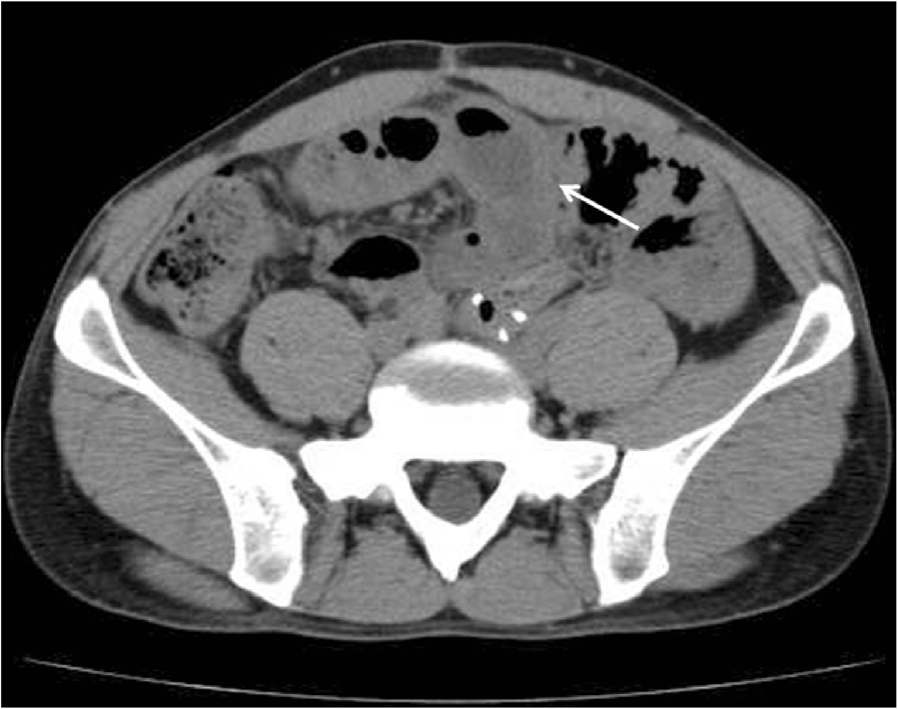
Abdominal computed tomography shows a dilated and filled small intestine enveloped by a thickened membrane (
